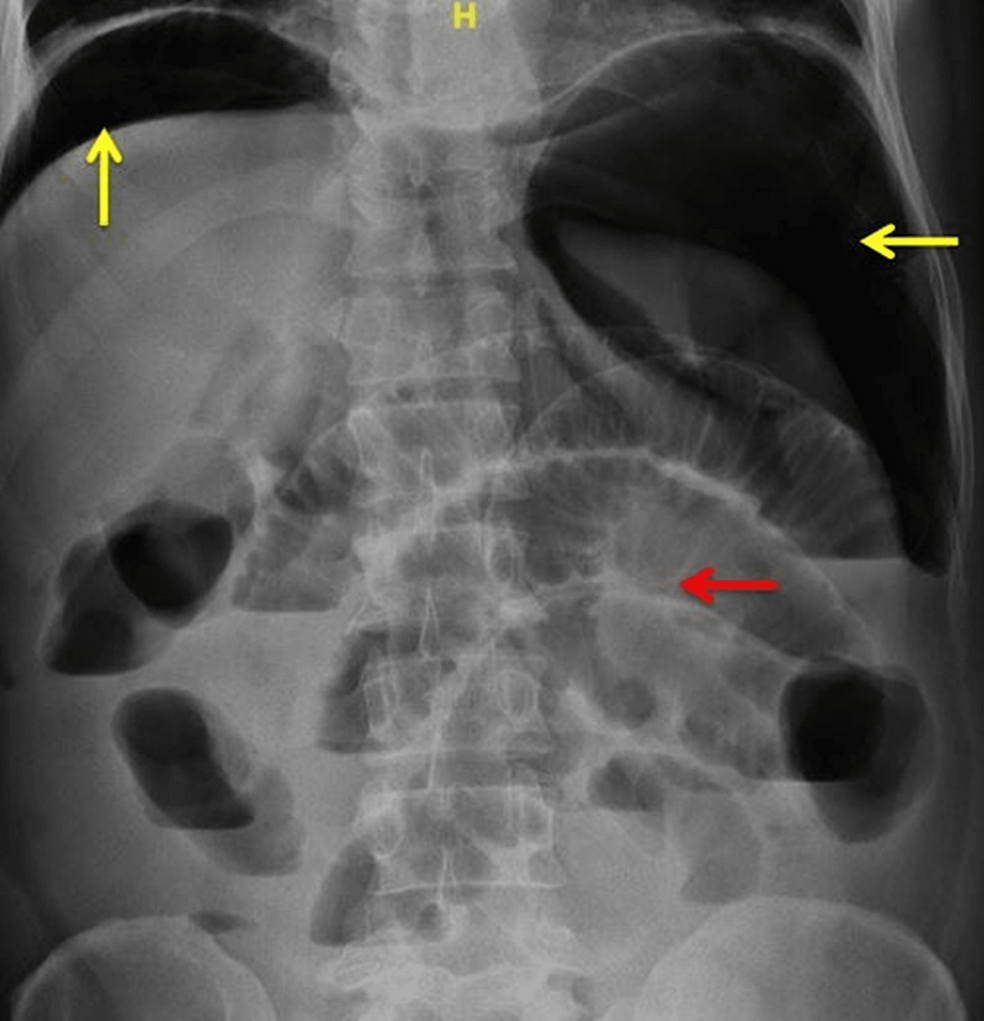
X-ray of the abdomen showing massive pneumoperitoneum (yellow arrows) and dilated small bowel loops with valvulae conniventes (red arrow).
